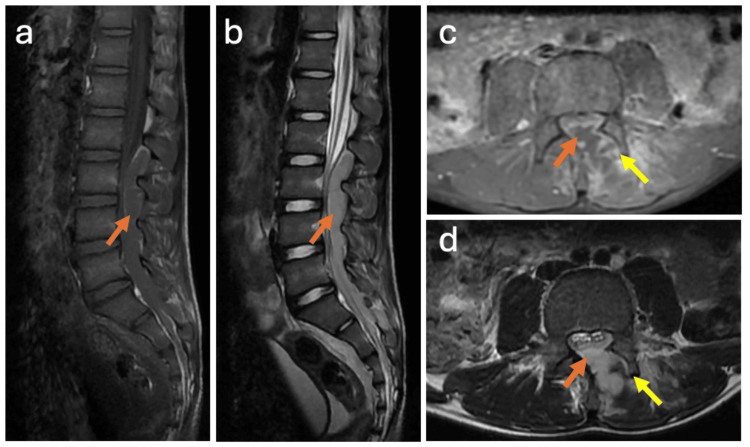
MRI of the lumbosacral spine demonstrating a posterior SEA arising from septic arthritis of the left L3-L4 facet joint (Case 1). Sagittal T1-weighted (a) and T2-weighted (b) images show a posterior epidural abscess extending from L2 to S2 (orange arrow), resulting in significant compression of the thecal sac and cauda equina nerve roots. Axial fat-suppressed contrast-enhanced T1-weighted images (c) demonstrate enhancement of the left L3-L4 facet joint (yellow arrow), the epidural collection (orange arrow), and the left paraspinal region. Axial T2-weighted images (d) show joint space widening and effusion of the left L3-L4 facet joint (yellow arrow) with epidural extension (orange arrow) and paraspinal muscle edema. SEA, spinal epidural abscess; MRI, magnetic resonance imaging
